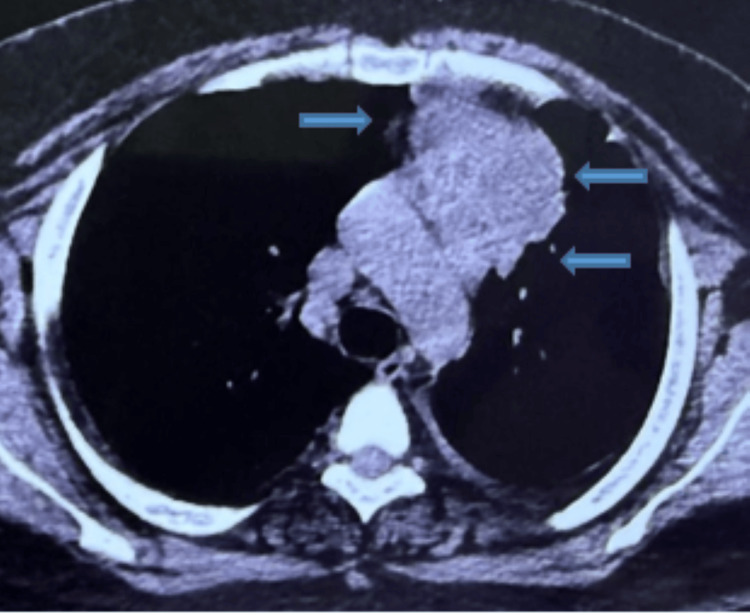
HRCT chest at two months demonstrating reduction in size and necrotic component of the mediastinal mass, confirming early radiologic response to ATT HRCT, high-resolution computed tomography; ATT, antitubercular therapy
